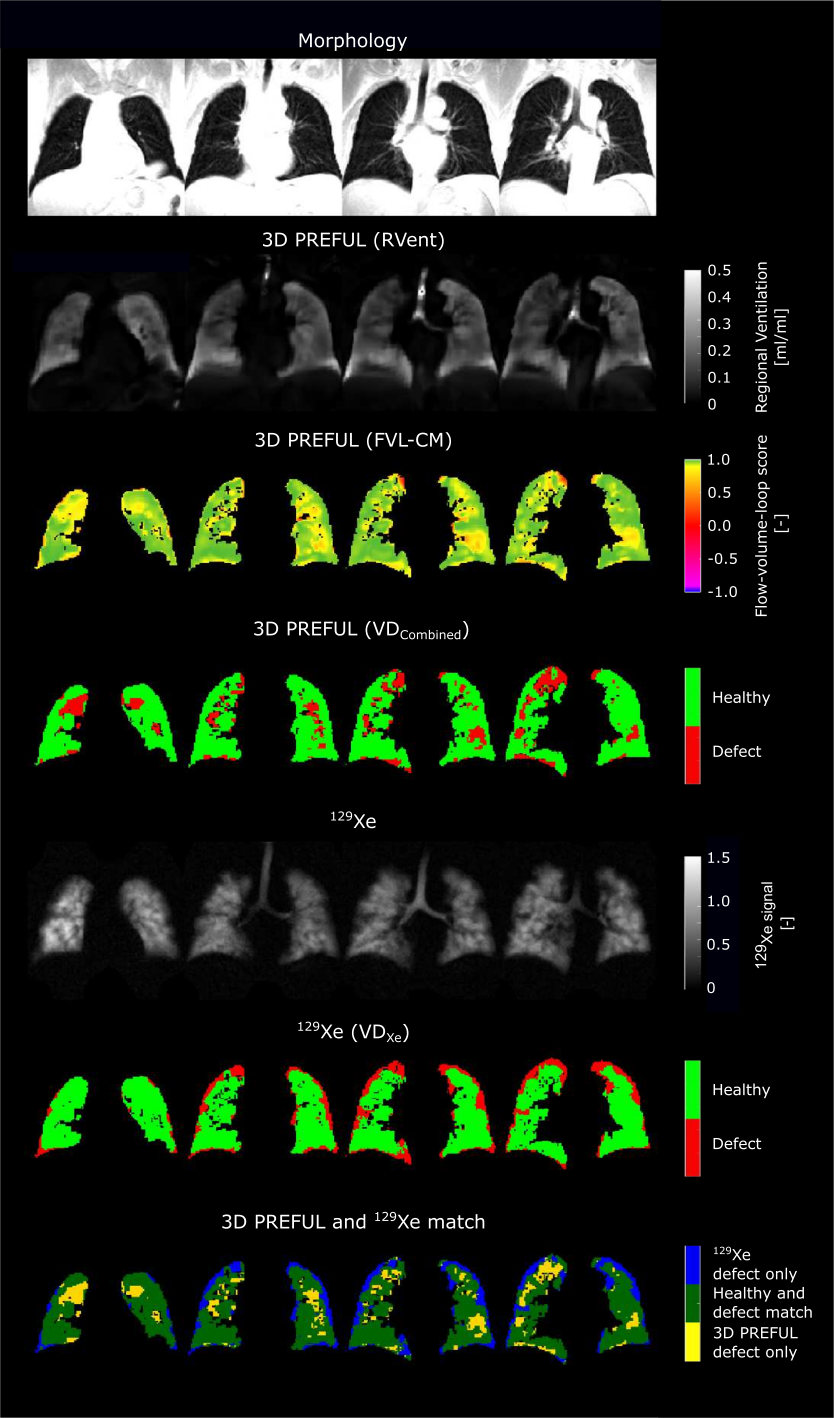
Exemplary morphological images (1st row) and respective ventilation parameter maps of a COPD patient (64-year-old male, FEV_1_ = 35% pred., FVC = 88% pred., GOLD III) by 3D PREFUL MRI (2nd–4th row, static RVent and dynamic flow-volume loop based parameter maps and combination of them) and ^129^Xe MRI (5th and 6th row), as well as spatial agreement of VD maps of both techniques (7th row). In all evaluated slices, the Sørensen–Dice coefficients were 0.17 in defect and 0.83 in healthy areas, which resulted in a total spatial overlap of 72.4%. Note the matched VDs and healthy areas (dark green) in both methods, along with minor differences in blue and yellow
